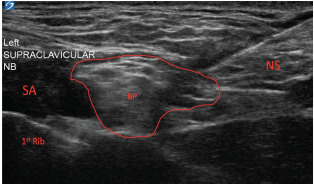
Ultrasound video still of supraclavicular brachial plexus block, with the brachial plexus sheath outlined in red. *SA*, subclavian artery; *BP*, supraclavicular brachial plexus; *NS*, needle shaft.
